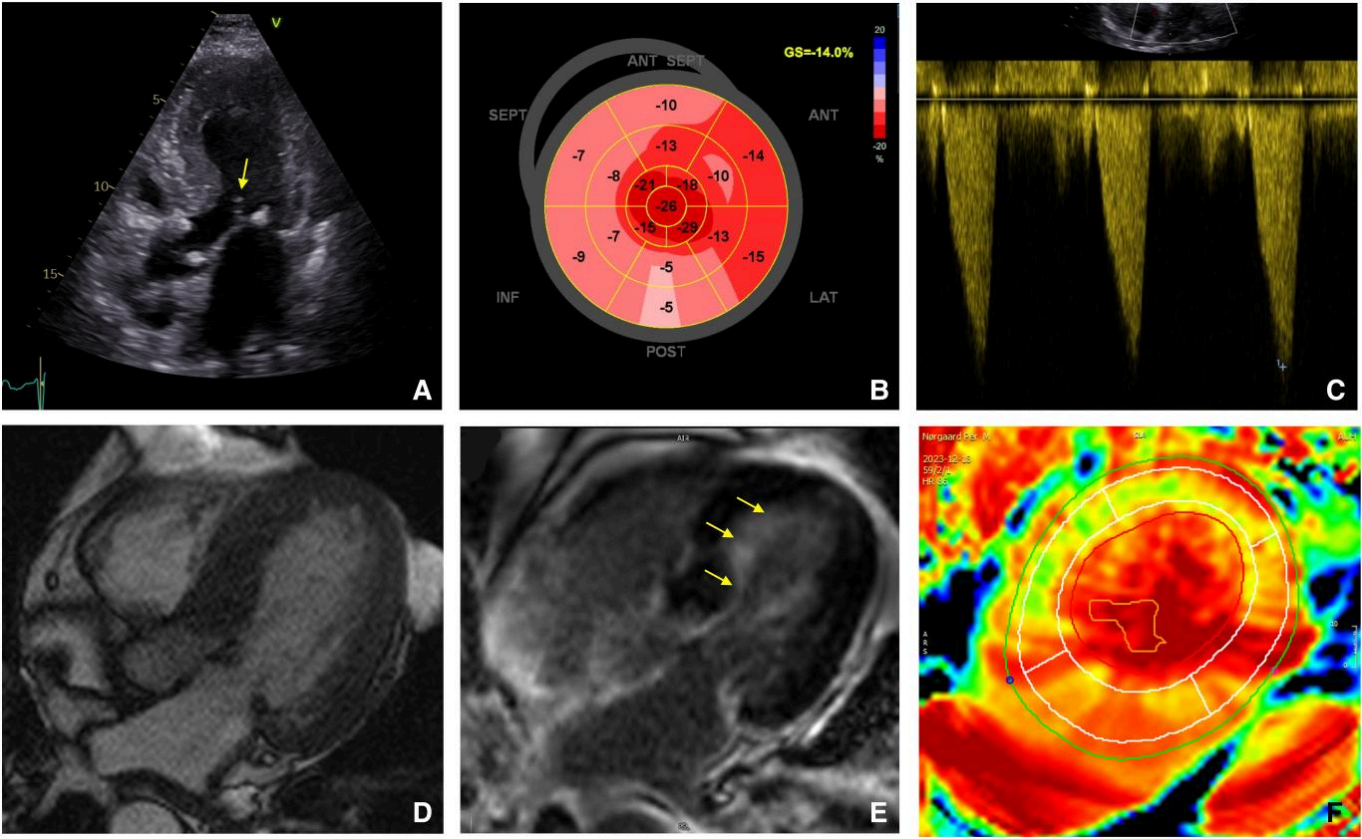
Imaging. (*A–C*) Echocardiography images: (*A*) apical five-chamber view displaying systolic anterior motion of the mitral valve (arrow). (*B*) Bull’s-eye map of the left ventricular global longitudinal strain pattern with relative apical sparing. (*C*) Continuous wave Doppler showing typical dagger-shaped signal of left ventricular outflow tract gradient. (*D–F*) Cardiac magnetic resonance images. (*D*) Cine Cardiac magnetic resonance image showing asymmetrical left ventricular hypertrophy. (*E*) Inversion recovery sequence showing late gadolinium enhancement with subendocardial late gadolinium enhancement pattern (yellow arrows). (*F*) Extracellular volume map.
